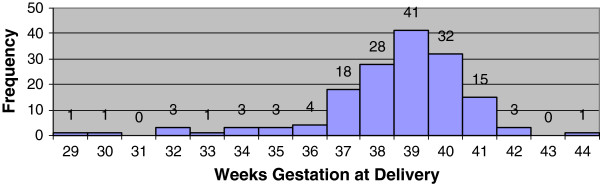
Distribution of gestational age in weeks at delivery by best obstetric estimate (ultrasound confirmed menstrual dates).
